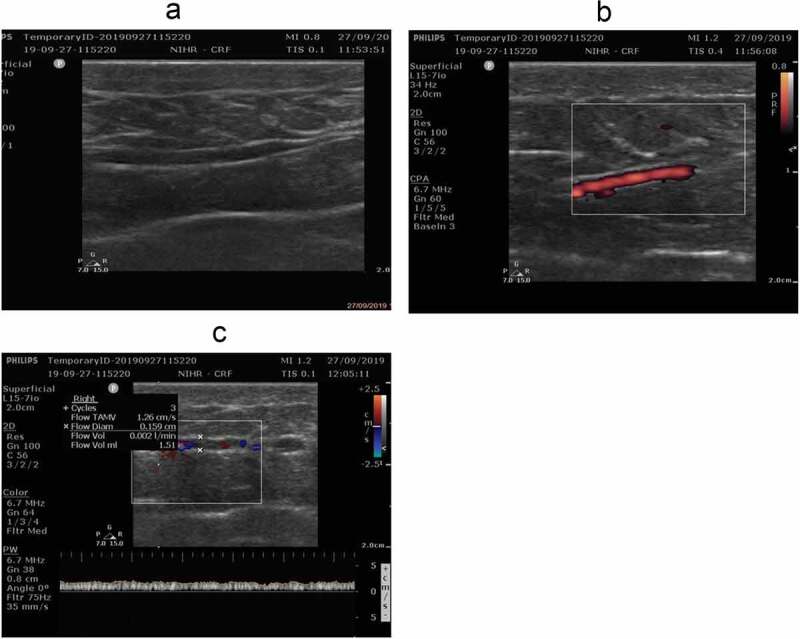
Representative images from the workflow of obtaining an ATBF measurement using Doppler ultrasound. Identification of a suitable subcutaneous adipose tissue vein (a), confirmation of flow signal in colour power angio (PCA) mode (b), followed by measurement of vessel diameter and calculation of ATBF (c)
